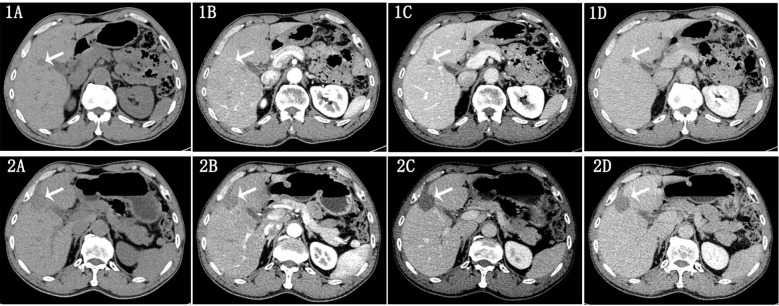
Male, 58 years old, diagnosed with HCC in liver segments S4/5 via clinical/pathological biopsy. Images 1A–D show contrast-enhanced CT scans 1 week before ablation therapy: Image 1A: Non-contrast scan shows a hypodense lesion. Image 1B: Arterial phase reveals mild heterogeneous enhancement. Image 1C: Venous phase demonstrates washout of contrast. Image 1D: Delayed phase shows further decrease in enhancement. (A–D) show follow-up contrast-enhanced CT scans 18 weeks post-ablation therapy: (A) Non-contrast scan shows a hypodense lesion. (B) Arterial phase reveals mild peripheral enhancement. (C, D) Venous and delayed phases show decreased enhancement. Conclusion: Incomplete resolution of the lesion after ablation therapy.
